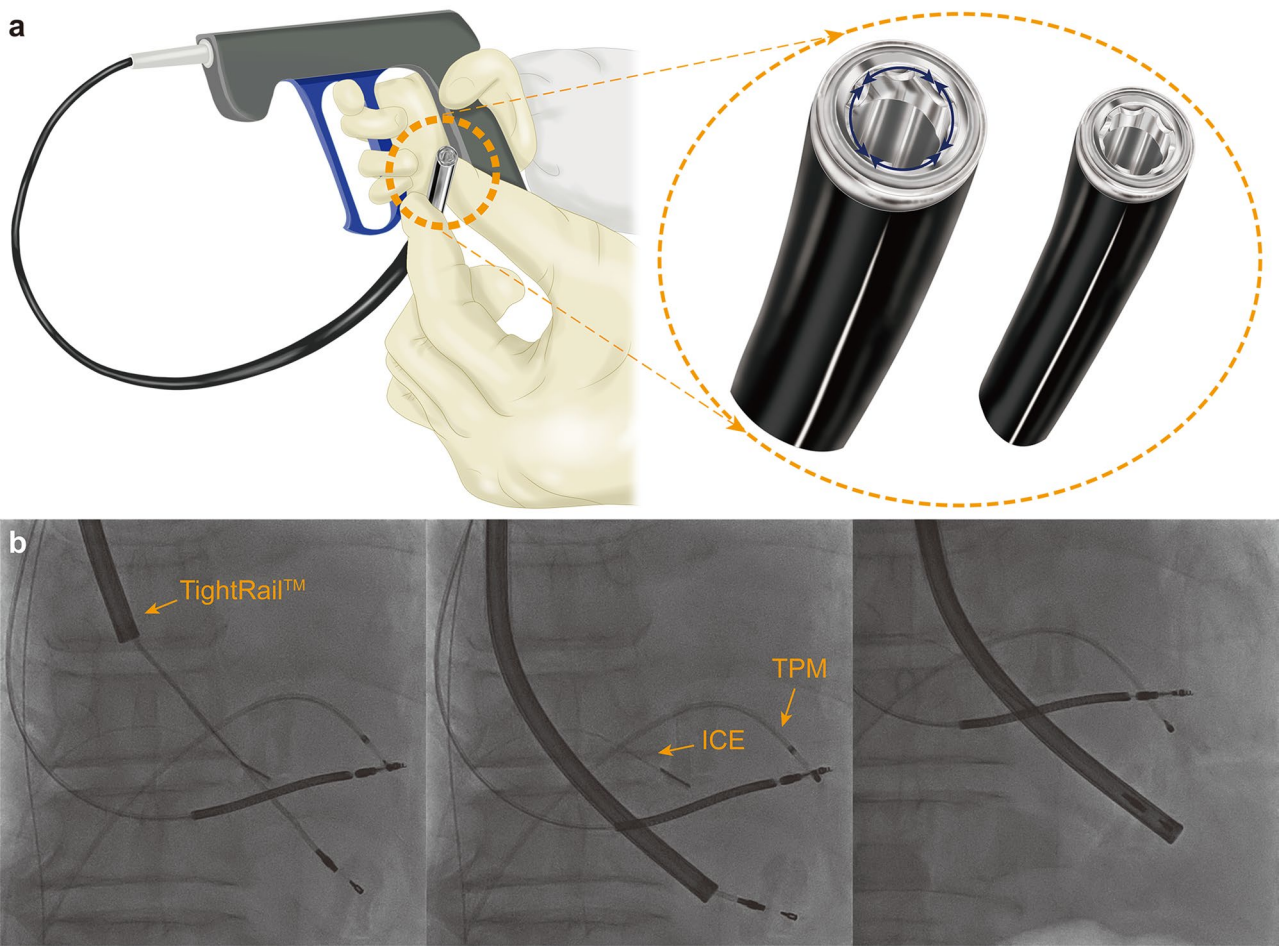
TightRail mechanical dilator sheath with bidirectionally rotating blade (a) and serial fluoroscopic image showing TLE procedure using TightRail sheath under ICE and temporary pacing wire backup (b). *ICE* intracardiac echocardiography probe, *TLE* transvenous lead extraction, *TPM* temporary pacemaker.
